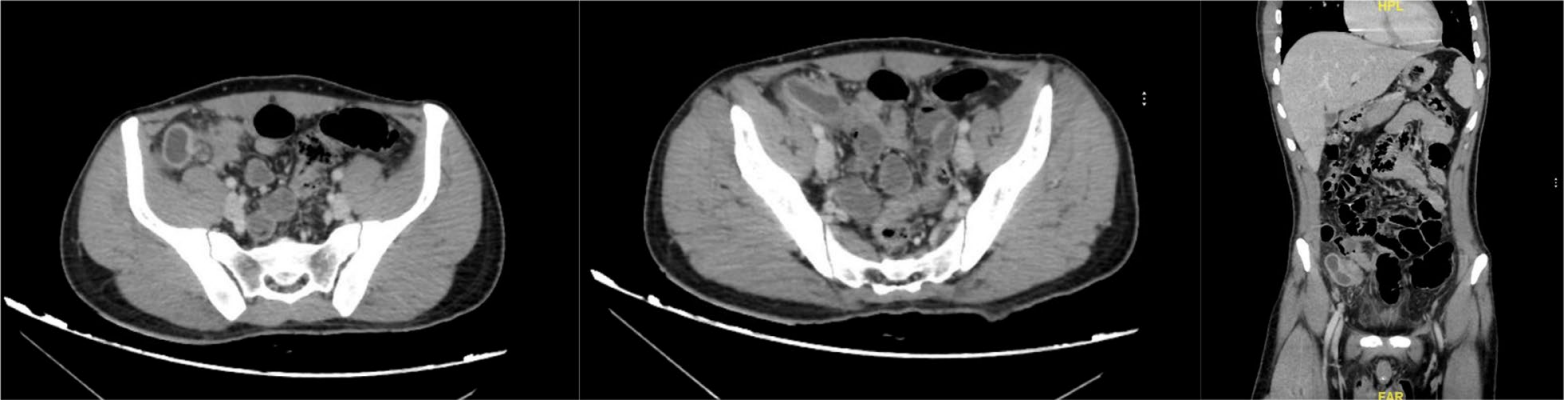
Axial, coronal CT appearance of the appendix
